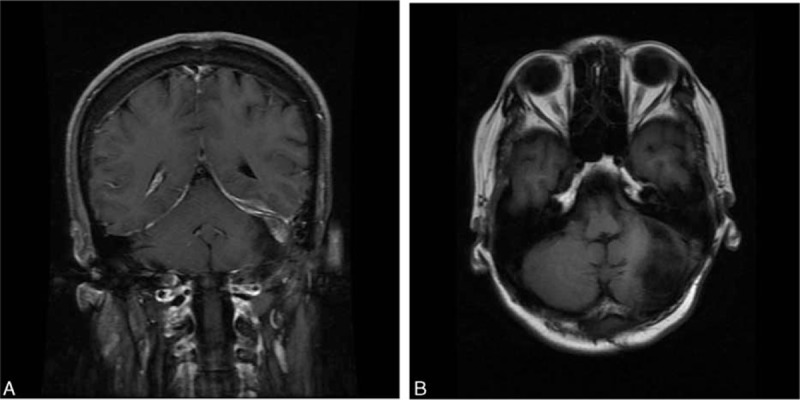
Half a year after the surgery, a MRI was performed, showing no recurrence.
